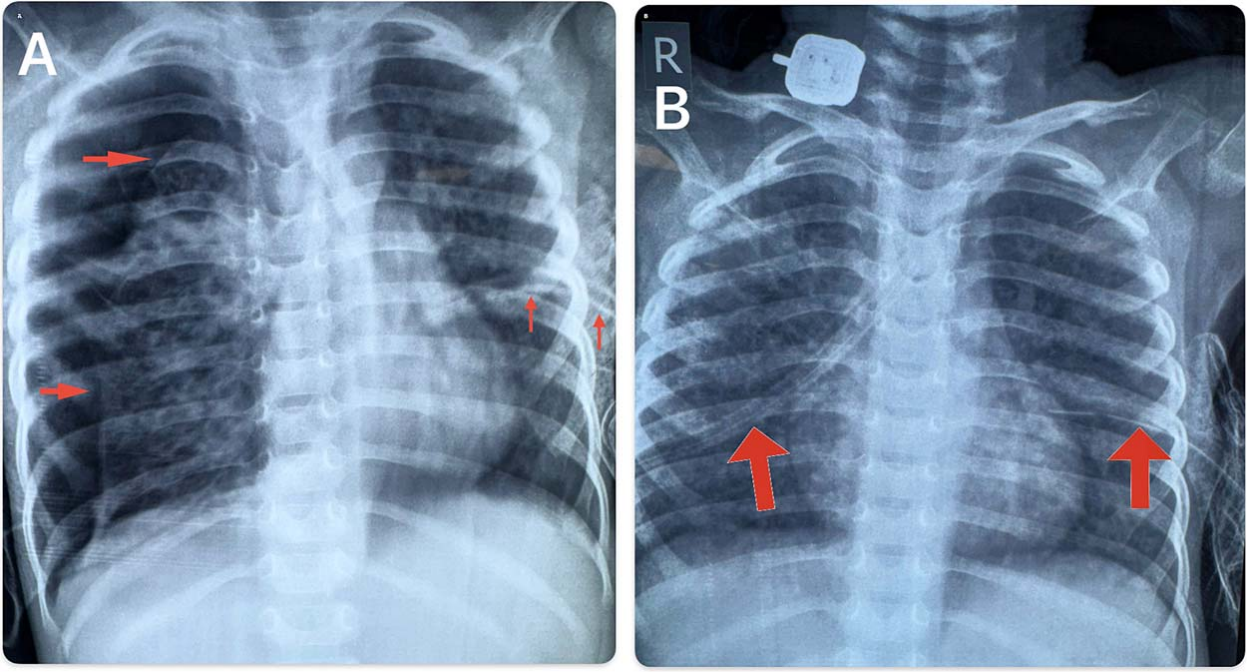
(A-B): Combined chest X-rays. (A) Chest X-ray showing a left-sided intercostal chest drain (ICD) in situ with a right-sided pneumothorax. (B) Chest X-ray after ICD insertion showing lung re-expansion with resolving pneumothorax.
